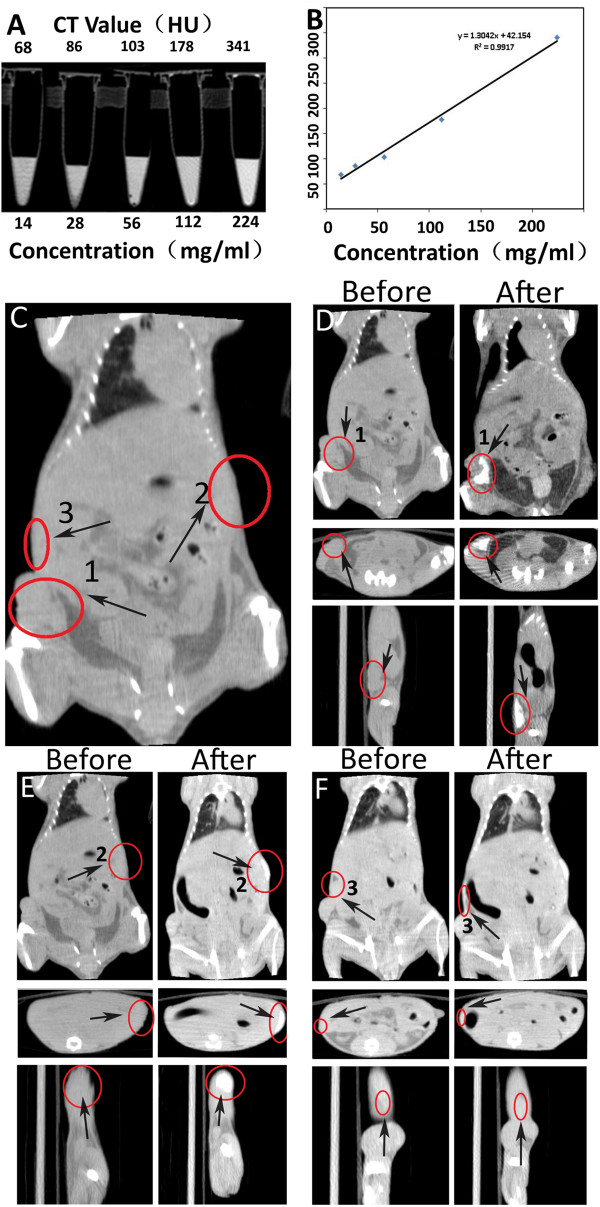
AuNCs@SiO_2_-FA nanoprobes for CT imaging. (A) In vitro CT images of AuNCs@SiO_2_-FA in 0.01 M PBS. The concentration (mg/mL) in each sample is provided at the bottom of the respective images; (B) CT attenuation (HU) plot of AuNCs@SiO_2_-FA at various concentrations in the range from 14 to 226 mg/ml. In vivo CT images of nude models before and after tail vein and subcutaneous local injection: (C) showed the two different sites and the control site, named area-1(tumor site), area-2(back site), area-3 (soft tissue), respectively; (D) and (E) showed the CT images before and after tail vein and subcutaneous injection of area-1 and area-2 respectively. (F) area-3 soft tissue was selected as a control to area-1 and area-2.
